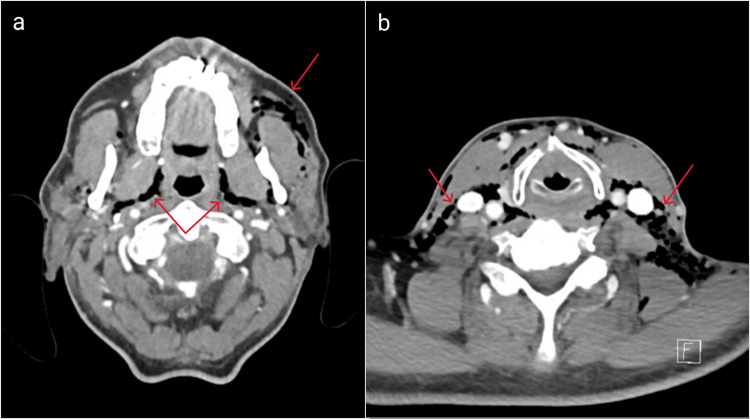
Multiple CT scan views of the neck area: (a) emphysema surrounding the lower left molar region and the floor of the mouth and (b) subcutaneous emphysema descending on the neck CT: computed tomography
